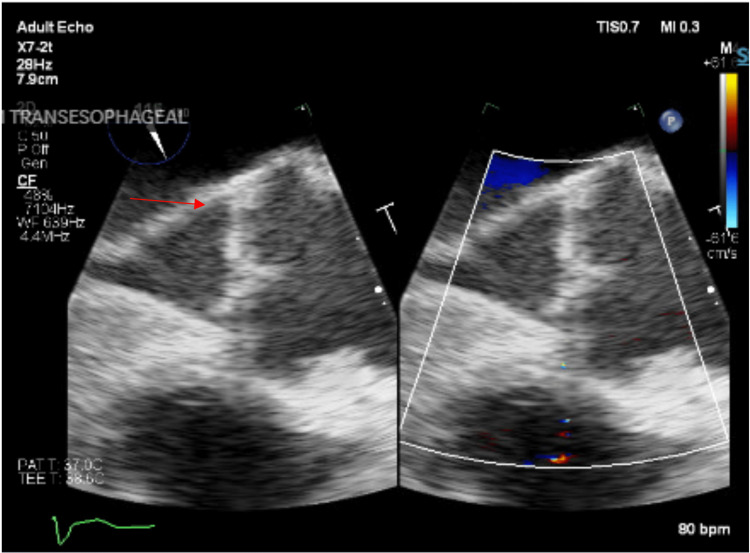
Transesophageal echocardiography with doppler. A small, loosely organized, mobile vegetation (red arrow) on the aortic valve, as characterized in Figure 3
